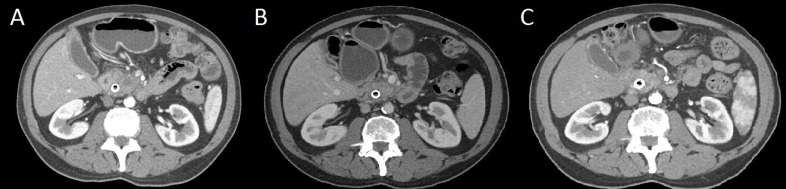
A. Pre-treatment computed tomography (CT) scan demonstrating ill-defined infiltrative mass measuring 2.7 cm x 5.0 cm. B. CT following 6 doses of FOLFIRINOX chemotherapy showing that the mass involving the head and uncinate process of the pancreas is difficult to define and measure but appears slightly less bulky as compared to the prior examination. C. 6-weeks post-SBRT CT scan reveals interval decrease in infiltrative pancreatic head mass.
